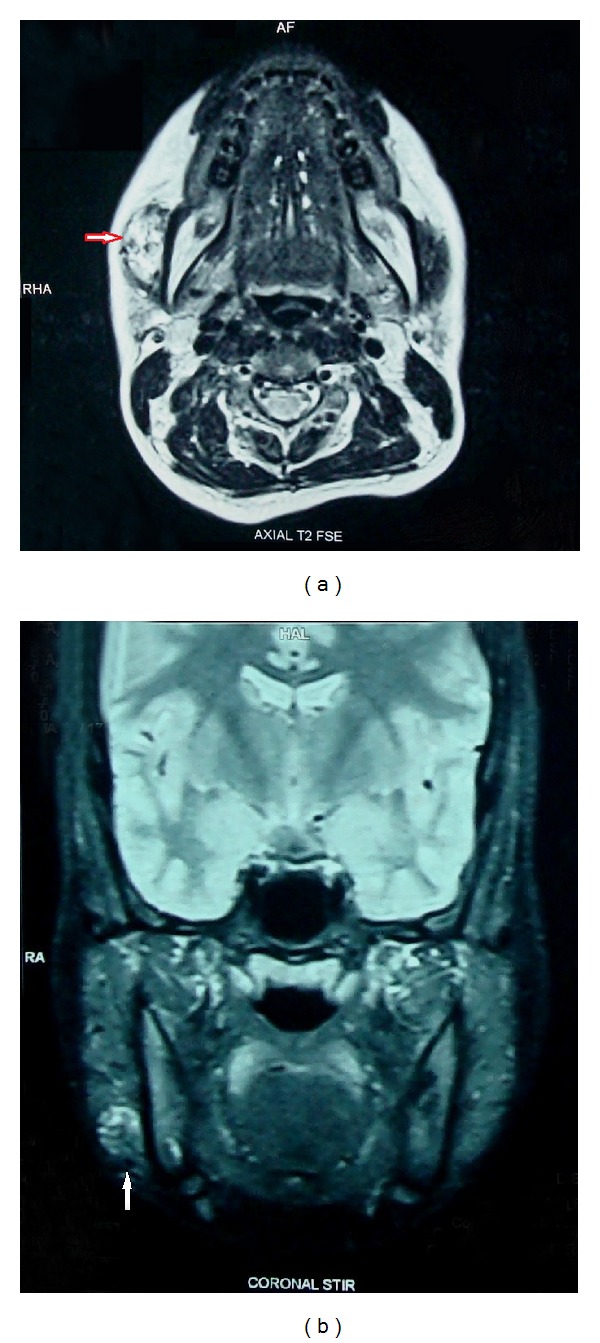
(a) T2 weighted axial view, MRI showing mixed hypo and hyperintense mass, along the anteroinferior aspect of the right masseter. (b) T2 weighted, fat suppressed coronal view of the lesion in right masseter.
